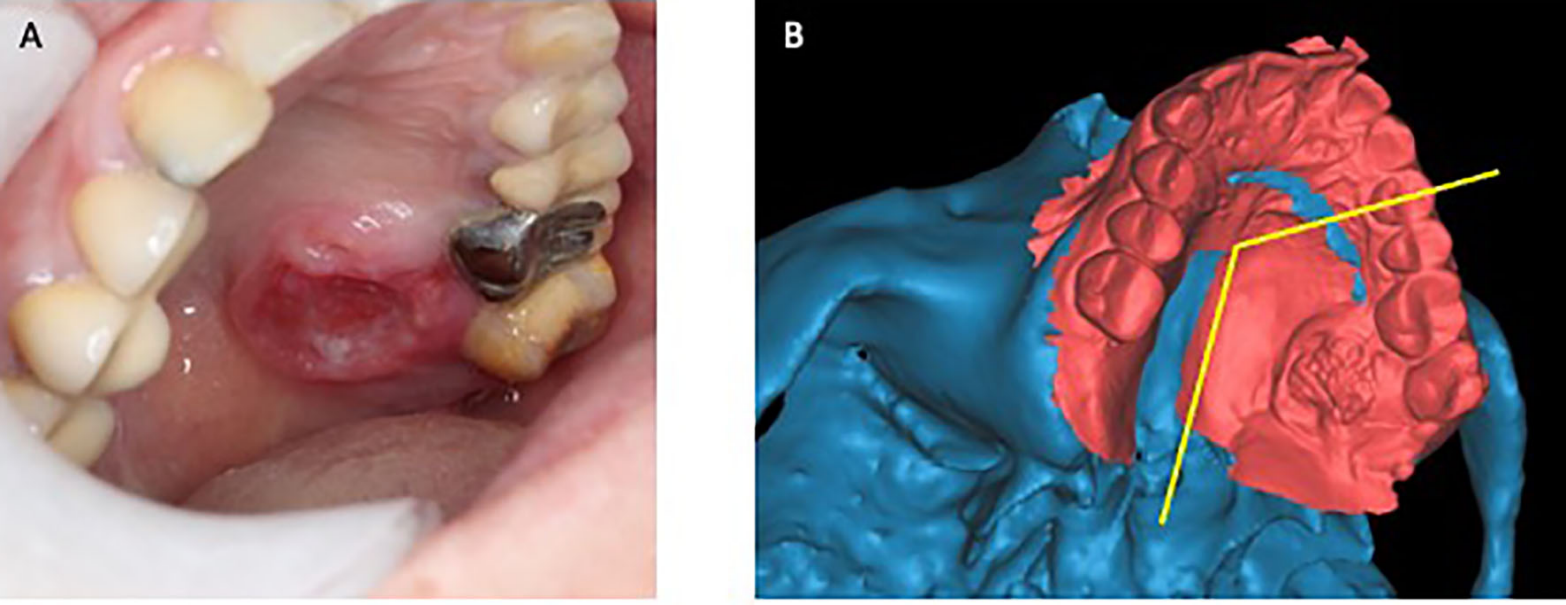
Superimposing intraoral scan to CT data for designing of surgical margin. (A) Clinical photo of a patient with squamous cell carcinoma at left palate. (B) Intraoral scan superimposed to the digital model built from CT scan. (Yellow line: planned resection margins).
